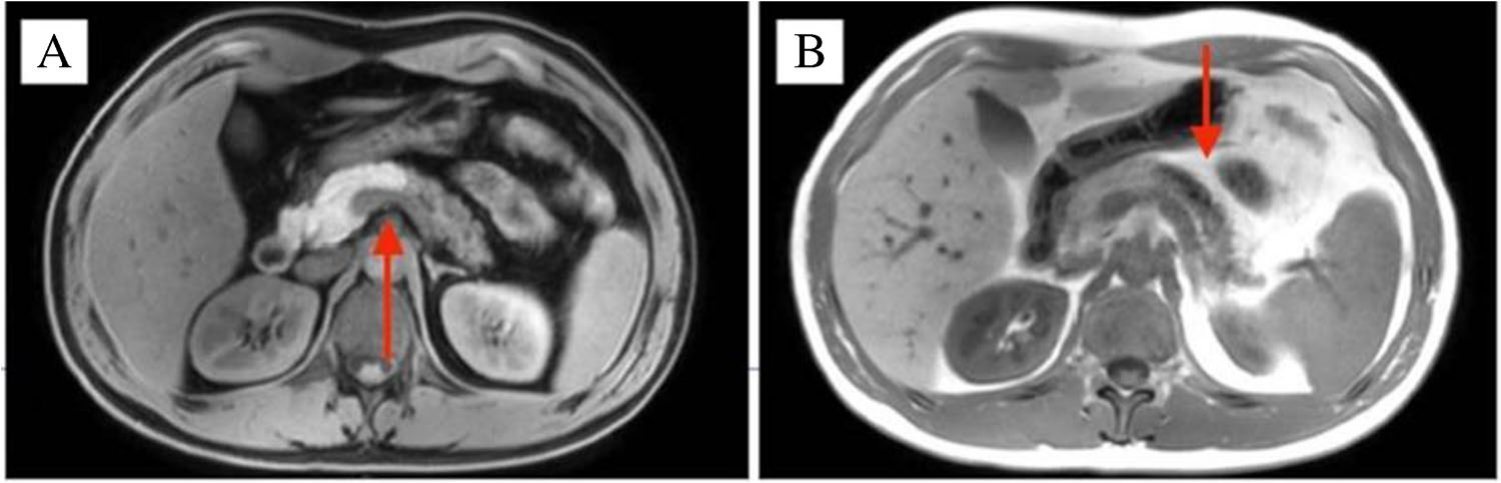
MRI scans demonstrating pancreatic main duct dilatation and adjacent pancreatic parenchymal atrophy and ductal dilatation: (A) axial T1-weighted image in the pre-contrast phase with hypoenhancement of the body and tail of pancreas and (B) T2-weighted in-phase scan with prominence of the dilated main pancreatic duct and mild increase in the enhancement of the surrounding parenchyma
